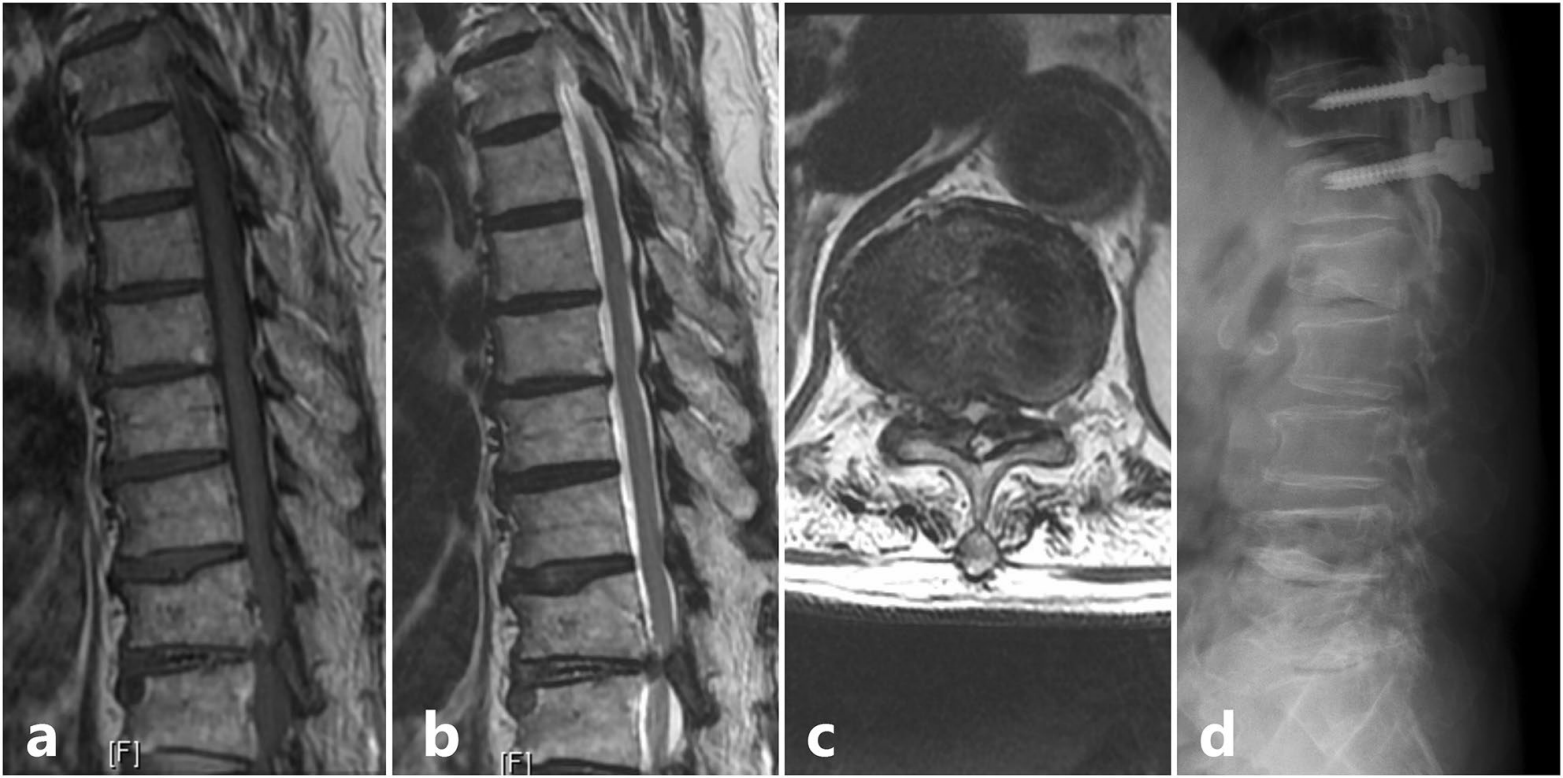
Scenario of a patient with solitary thoracic lesion who presented with category 2 signs; (a) Sagittal T1 weighted MRI showing an Ossified Ligamentum Flavum (OLF) at T11-T12 level; (b) Sagittal T2 weighted MRI; (c) Axial T2 weighted MRI showing the significant stenosis caused due to the OLF at T11-T12 level; (d) Post decompression and stabilization lateral view X-ray image.
